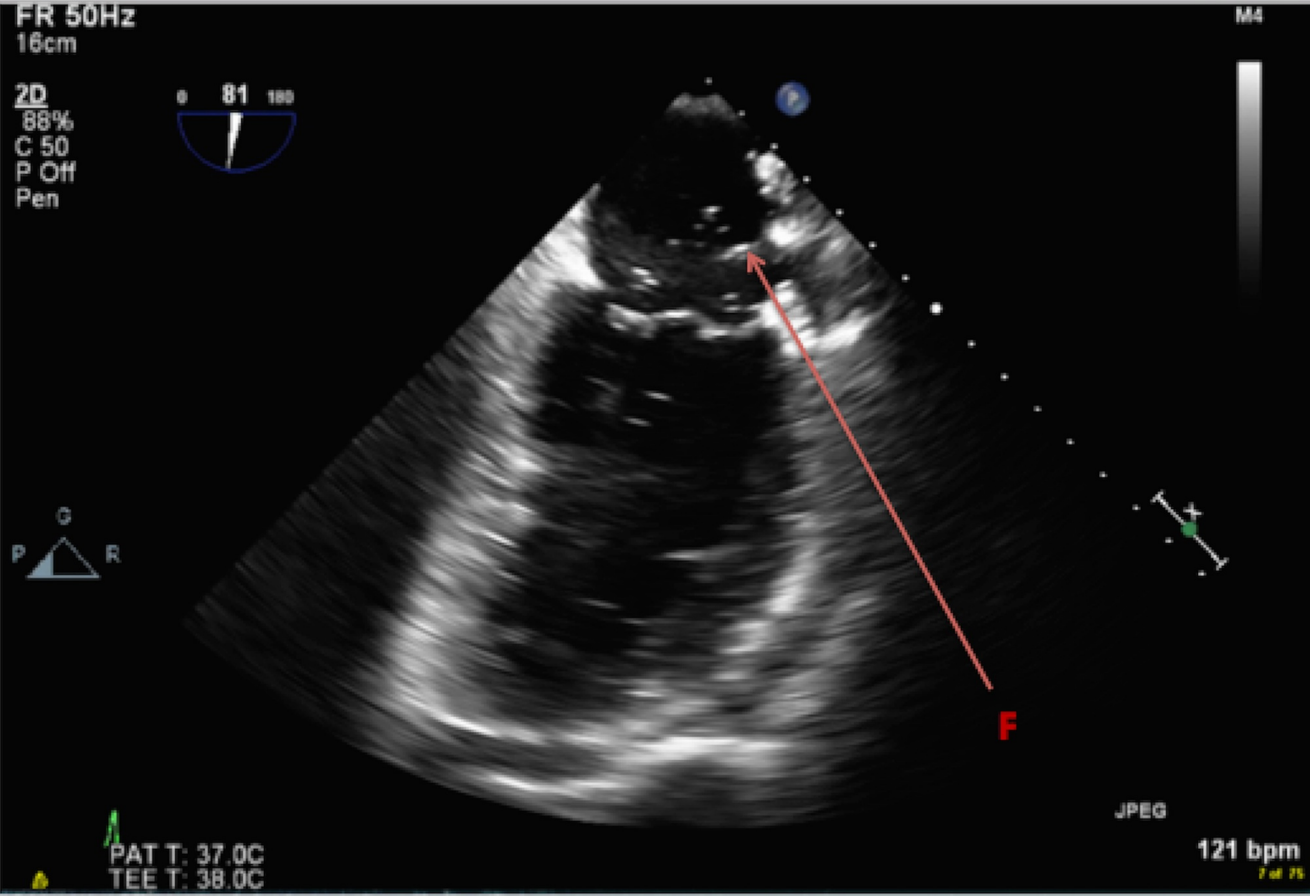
F: Transesophageal echocardiogram (TEE) demonstrating mediastinal mass extending into the left atrium from the left upper pulmonary vein
